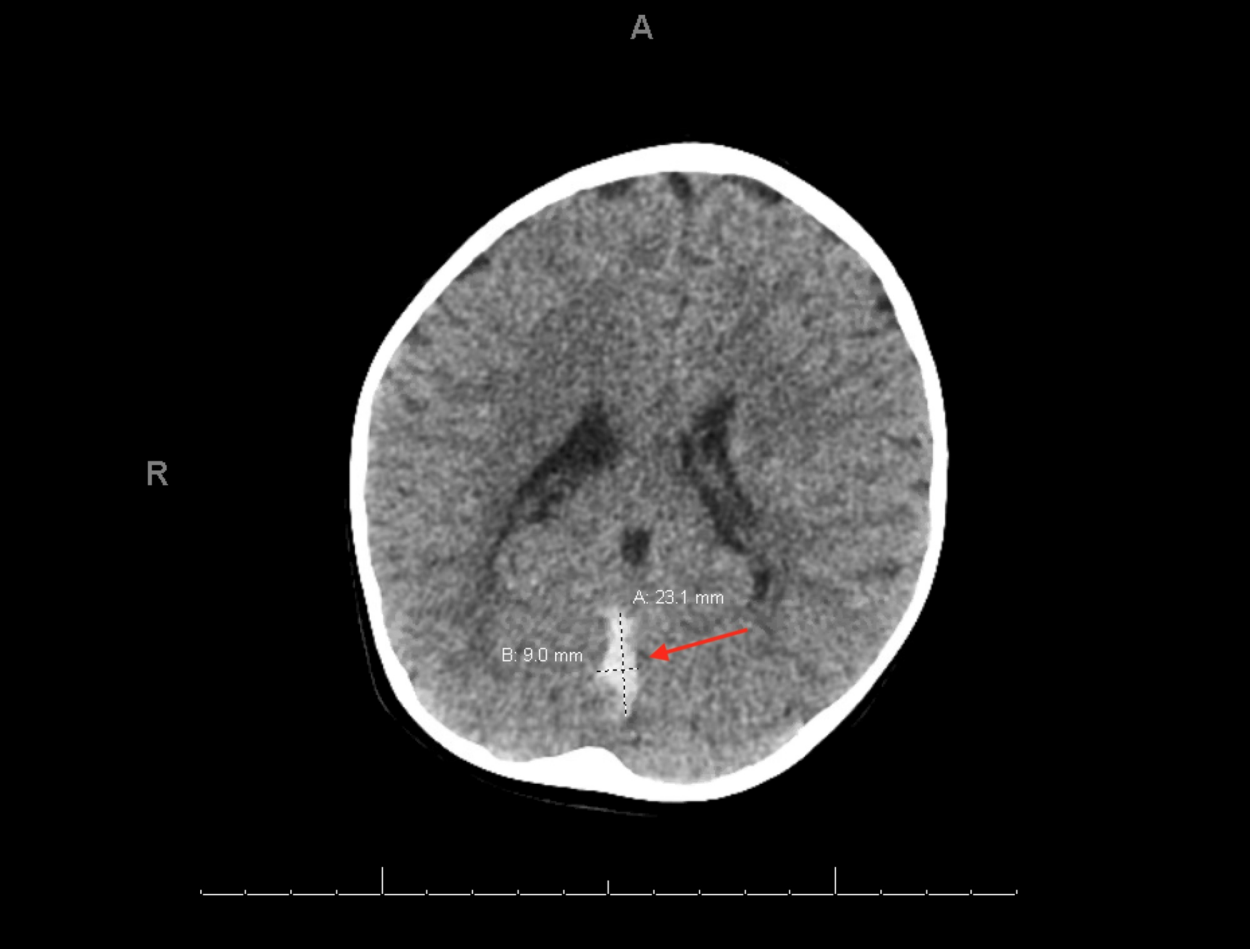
Brain CT scan showing hyperdense collection within the posterior interhemispheric region.
